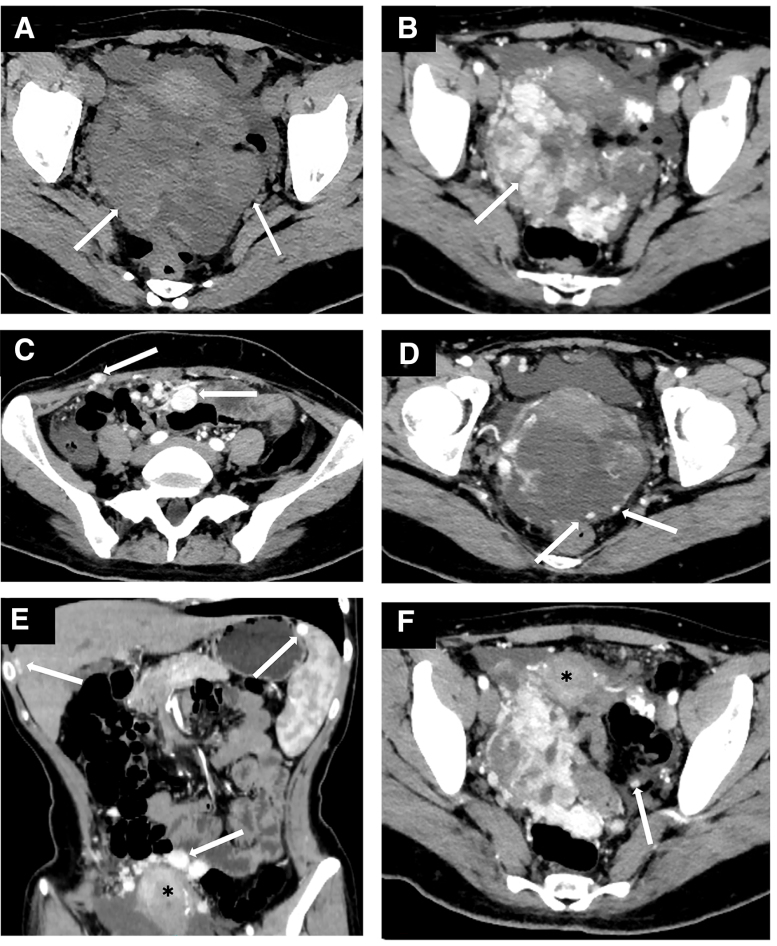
Abdominal CT image. (A) Non-contrast CT scan reveals bilateral adnexal masses. (B) Post-contrast imaging shows marked enhancement above the myometrium (black asterisk indicates uterus). Omentum (C), peritoneum (D), uterine serosal surface and hepatic/splenic capsule (E), sigmoid colon serosal surface (F) with multiple hyperenhancing nodules (white arrows indicate nodules). CT = computed tomography.
